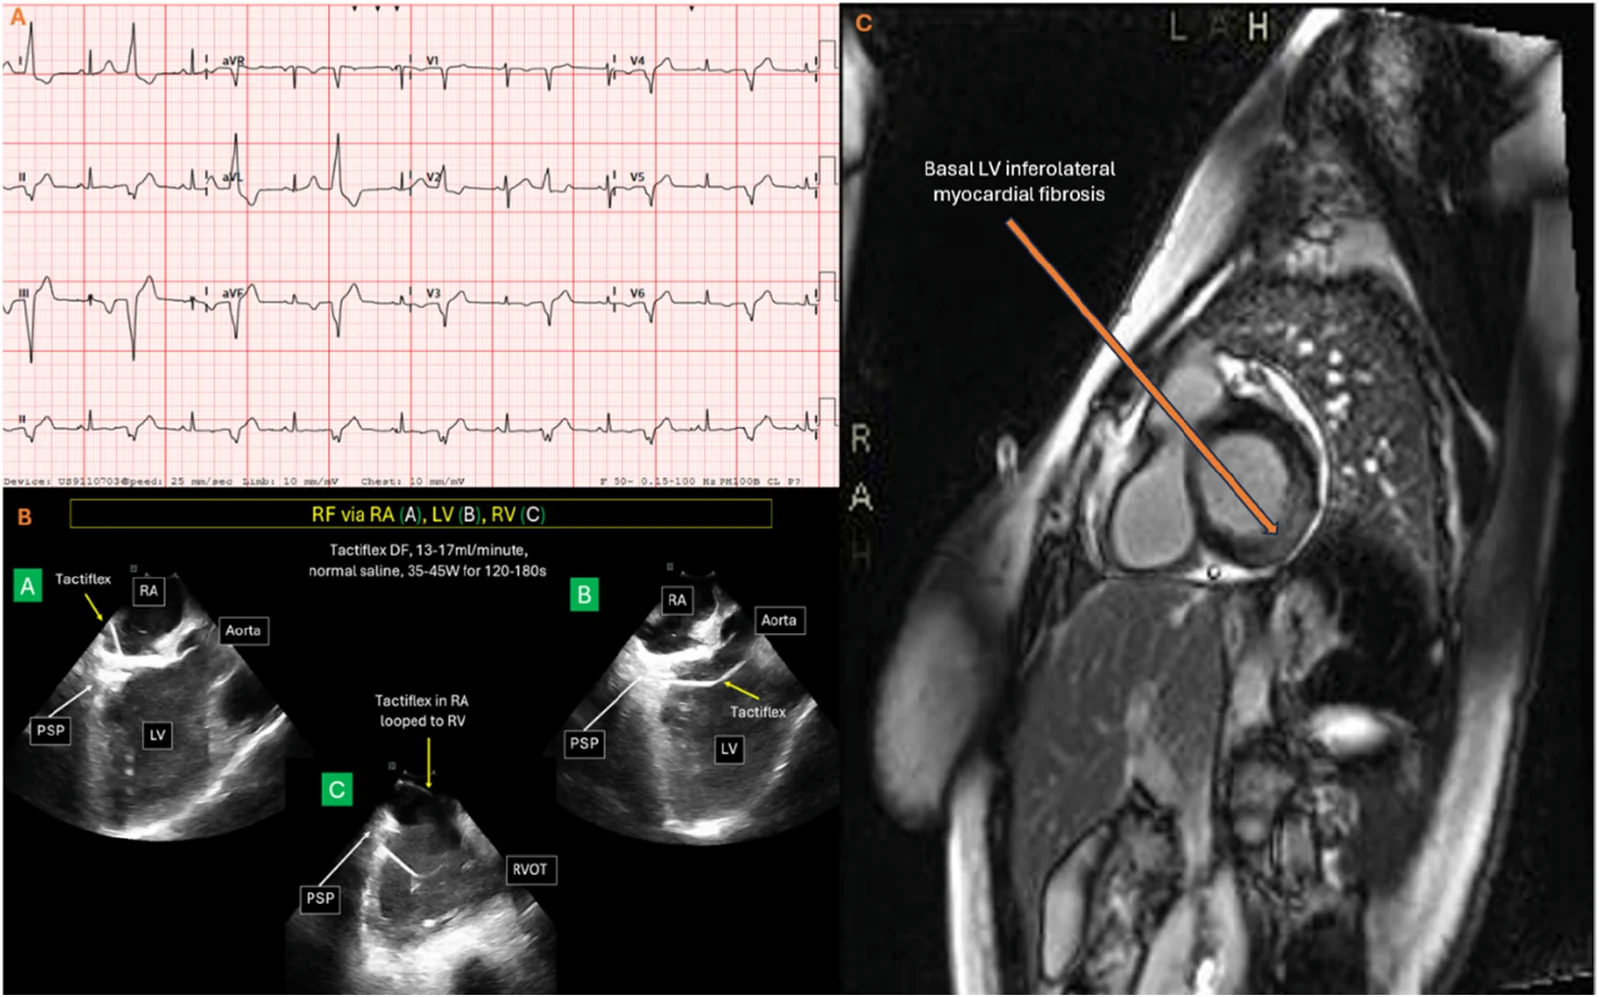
A. Left superior axis deviation with ‘reversed V2 lead pattern break’ and LBBB morphology in Lead V1 and short QRS duration of 86 ms. B. ICE imaging during targeted radiofrequency ablation within all three adjacent cardiac chambers (RA, RV, and LV). C. Cardiac MRI with late gadolinium enhancement demonstrating myocardial fibrosis at the basal LV inferolateral region.
